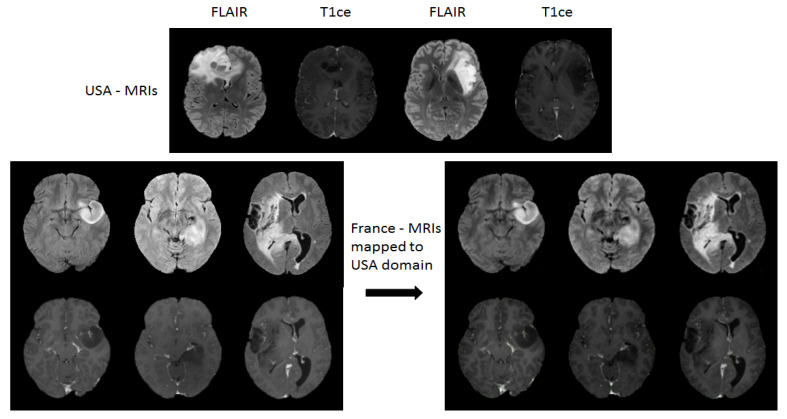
Visual inspection of image slices before and after the domain mappings. Top: Examples of FLAIR-MRIs and T1ce-MRIs from USA dataset. Bottom left: 3 original 2D slices of FLAIR-MRIs (1st row) and T1ce-MRIs (2nd row) from France dataset. Bottom right: Domain mapped 2D slices of FLAIR-MRIs (1st row) and slices from T1ce-MRIs (2nd row) from France to USA domain.
